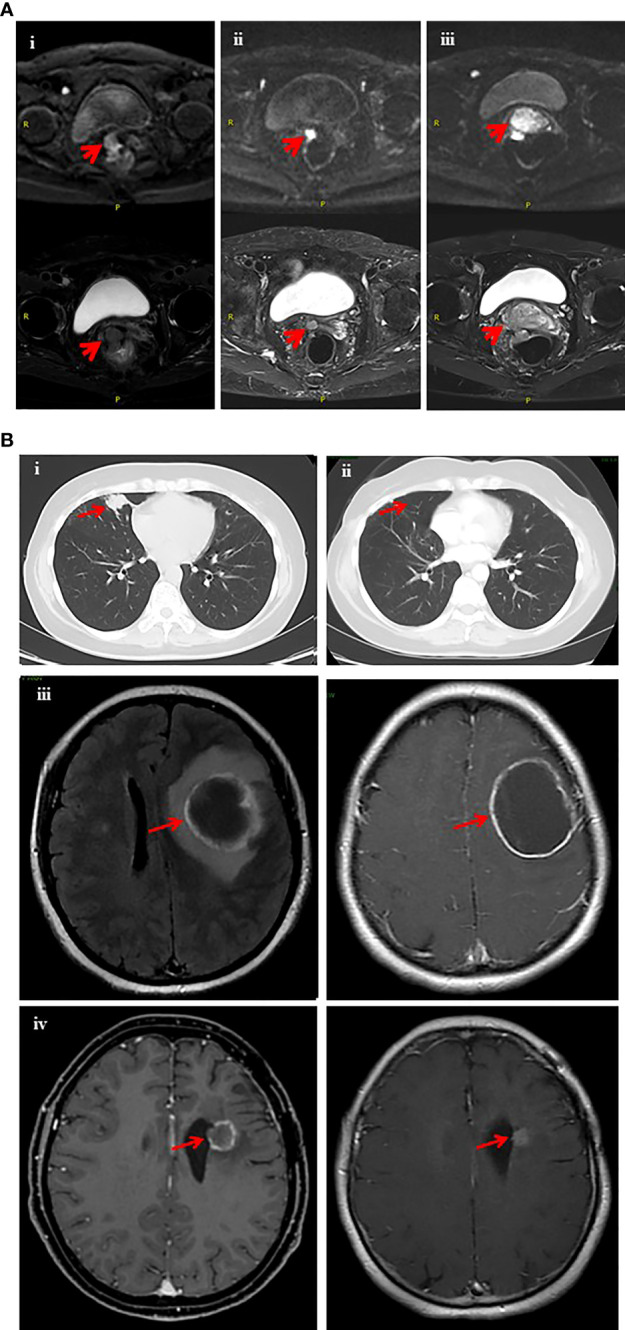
Imaging records of two patients who had *BRCA1/2* LGRs and benefited from PARP inhibitors. (A) Magnetic resonance imaging (MRI) scan of the pelvic before and after fluzoparib treatment (ovarian cancer case). The red arrows indicate tumor lesions. (i) The pelvic MRI scan on March 5, 2021 revealed tumor status before fluzoparib treatment (5.5*3.4 cm). (ii) Pelvic MRI on June 9, 2021 indicated a significant decrease in tumor size (1.9 *1.6 cm) after fluzoparib treatment. (iii) The pelvic MRI scan on August 10, 2021 revealed progression of tumor lesions (3.0*4.2 cm) after 3 months of discontinuation of fluzoparib. (B) Computed tomography CT scan of the chest and magnetic resonance imaging (MRI) scan of the brain (breast cancer case). The red arrows indicate tumor lesions. (i) Chest CT in May 2017 revealed a metastatic lesion (approximately 2.3*1.8 cm) in the middle lobe of the right lung. (ii) The patient received surgery in September 2017 and maintenance therapy with olaparib. Chest CT in October 2021 indicated pulmonary metastasis disappeared during maintenance therapy with olaparib. (iii) Brain MRI in August 2017 revealed a metastatic lesion with ring-like enhancement in the left frontal lobe with peri-tumoral edema. (iv) Brain MRI in October 2021 indicated a significant shrinkage in brain metastasis lesions during the maintenance therapy with olaparib.
